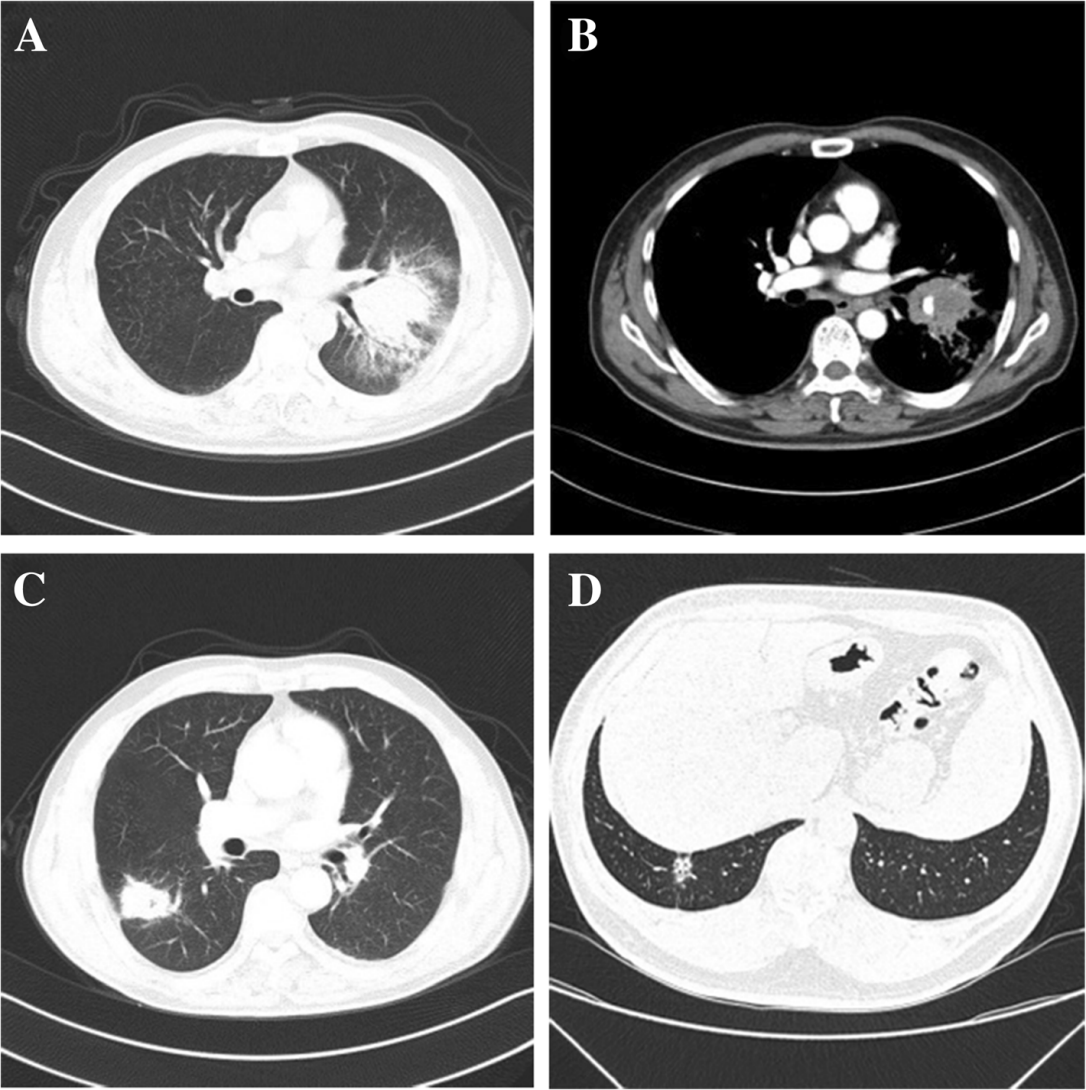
Thorax CT manifestations of OP cases. a, b OP presenting as a bulky consolidated mass with coarse margins and moderate enhancement in left upper lobe with hilum and mediastinal lymphadenectasis. c OP presenting as a mass-like lesion with coarse margins and pleural indentation, and low-density air is noted in the central in the right lower lobe. d OP presenting as a predominantly ground glass opacity, air bronchogram and pleural indentation in the right lower lobe
